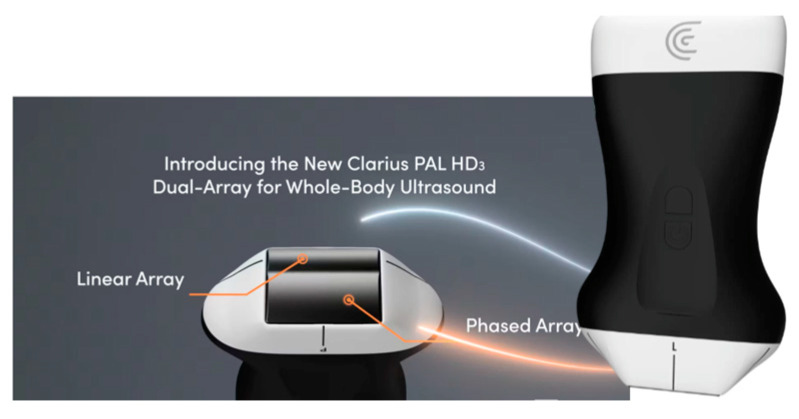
Clarius PAL HD3, adapted from (https://clarius.com/pal-dual-array-ultrasound) with permission publisher 2024 (accessed on 1 January 2024) [37].
